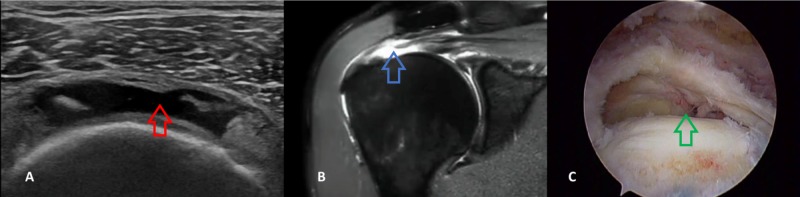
A 52-year-old male patient with a full-thickness (total) supraspinatus tear Longitudinal HRUSG image of the supraspinatus tendon reveals a hypoechoic discontinuity of the supraspinatus tendon (A: red arrow) extending from the articular to bursal surface. Note the cortical irregularity. STIR coronal MRI image of the shoulder joint reveals a full-thickness tear in the supraspinatus muscle with joint effusion (B: blue arrow). The arthroscopic image demonstrates a total full-thickness supraspinatus tear (C: green arrow). HRUSG - high-resolution ultrasonography; STIR - short TI Inversion Recovery
